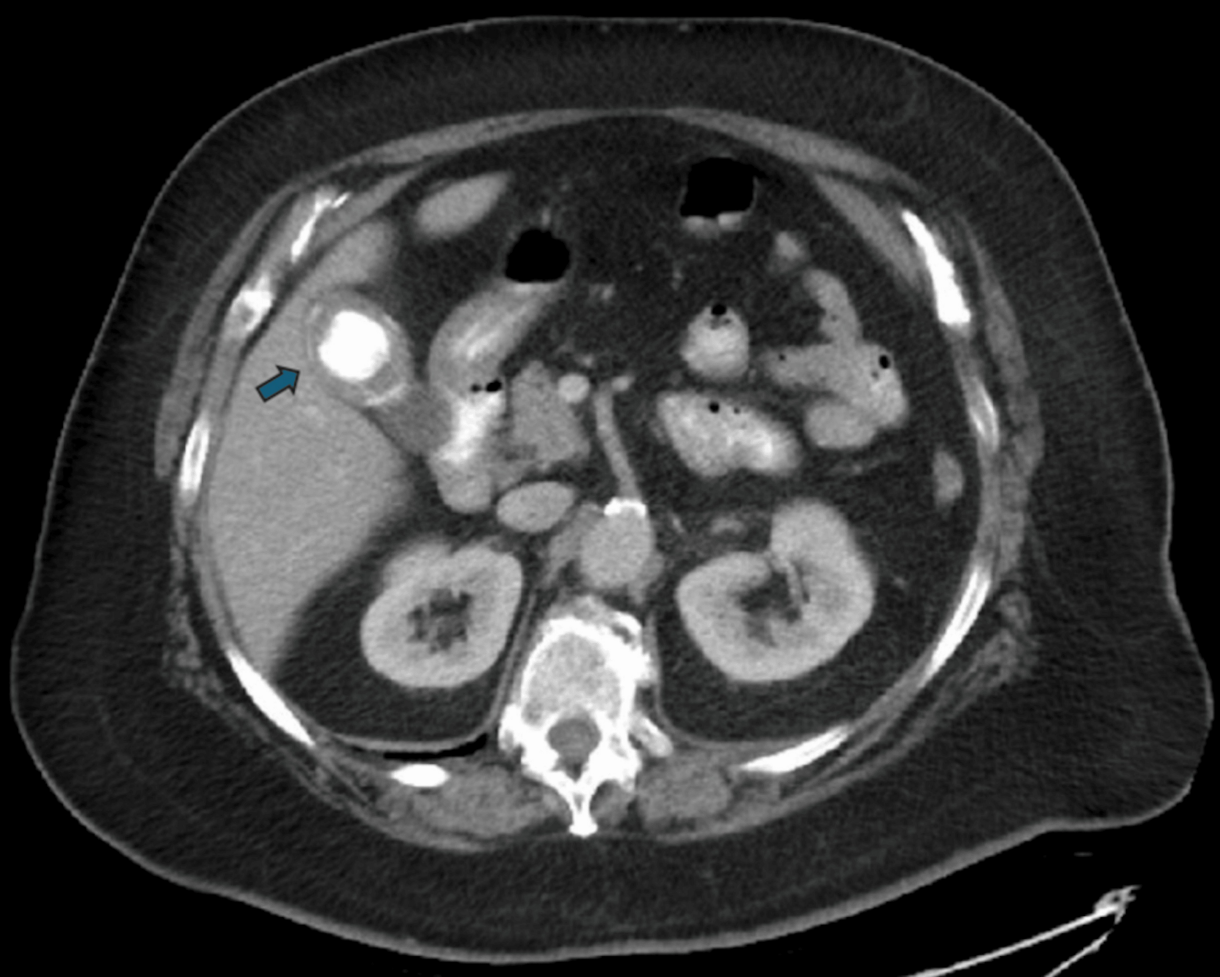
Historical CT scan demonstrating an identical calcified mass This image is from a CT scan performed six years prior to presentation to evaluate an ovarian cyst. It incidentally reveals a large calcified mass (blue arrow) within the gallbladder.
